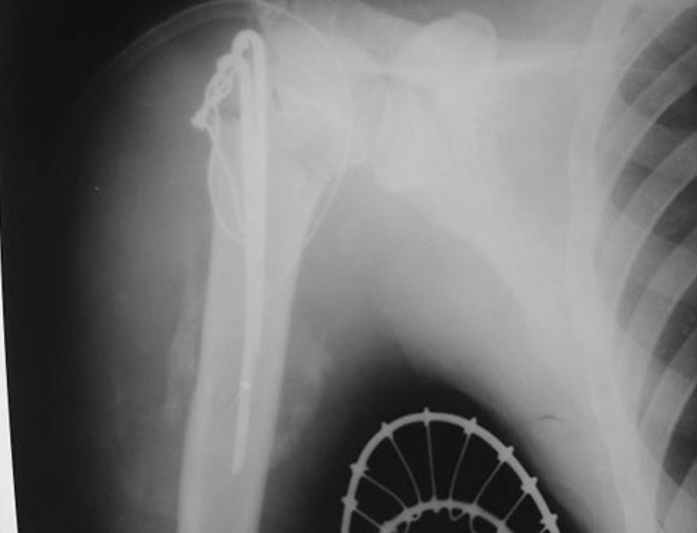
Post operative radiograph showing acceptable reduction
